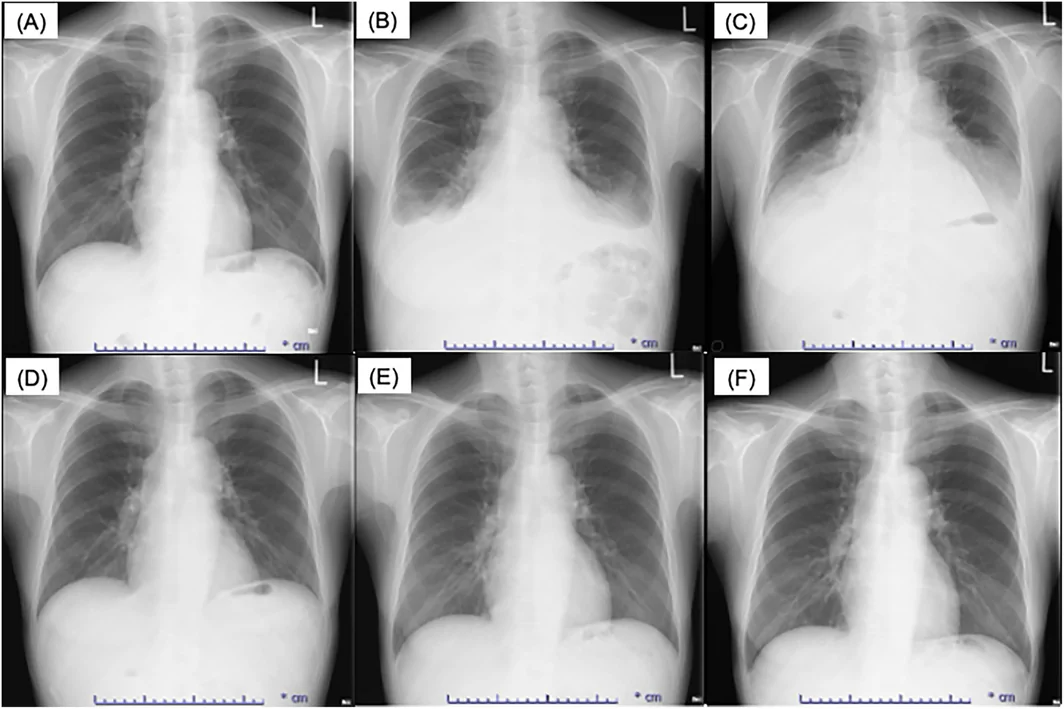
Serial chest radiographs during the clinical course. (A) At initiation of belzutifan therapy, showing normal cardiac size (CTR, 41.4%) without pleural effusion. (B) On the day of admission (27 days after belzutifan initiation), demonstrating marked cardiomegaly (CTR, 63.4%) and bilateral pleural effusions. (C) Hospital day 5, showing transient worsening of pulmonary congestion and pleural effusion despite initiation of heart failure therapy. (D) Hospital day 16, on the day of belzutifan rechallenge, demonstrating improvement in cardiomegaly (CTR, 47.3%). (E) Eighteen days after belzutifan rechallenge, showing no recurrence of cardiomegaly (CTR, 44.8%). (F) One day after discontinuation of belzutifan, showing normal cardiac size (CTR, 42.1%).
